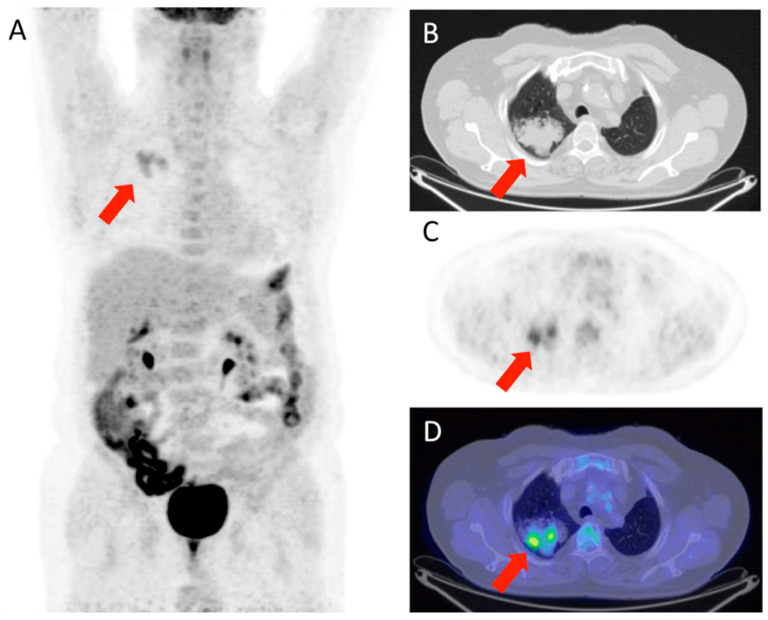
Patient with lung relapse. 18F-FDG PET/CT was interpreted as positive for 18F-FDG uptake as MIP (A), transaxial CT (B), PET (C), and fused (D) images showed 18F-FDG uptake described as focal (with two foci), deep, and inhomogeneous. The semiquantitative parameters were gSUVmax 3.6, MTV 17,41, pSUVmax 3.4, dSUVmax 1.18, MSD 2.87, and IDSD% 34.7%.
